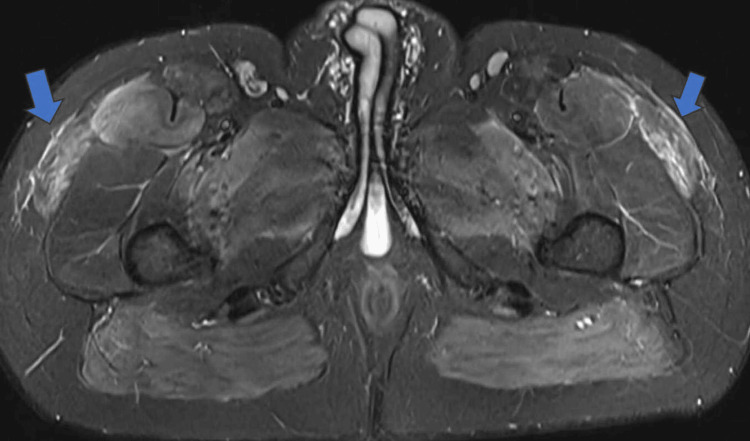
MRI scan of the thighs showing high signal intensity (blue arrows).
